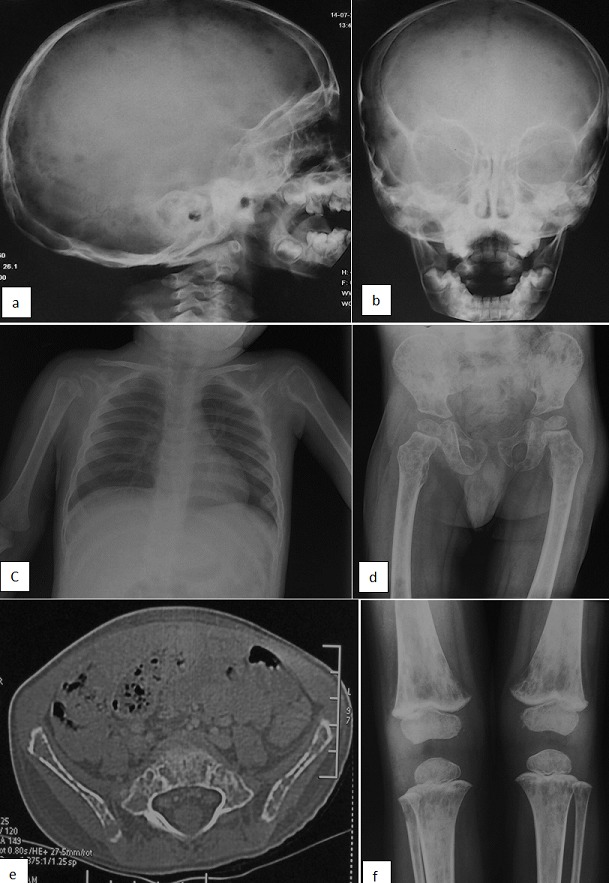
(A,B) multiple osteolytic lesions in the skull, humerus, pelvic bone, both femurs, and both tibias; Skull X-ray (front and profile): reveals multiple lytic lesions; (C) front chest X-ray revealed proximal metaphyseal humoral lytic lesion responsible for fracture; (D) X-ray reveals multiple osteolytic lesions in pelvic bone and both femurs; (E) lumbosacral and pelvis CT Scan revealed diffuse lytic lesions and permeative bony lesions in iliac bones; (F) X-ray of knee joints reveals metaphyseal lytic lesions in both distal femurs and proximal tibiae
